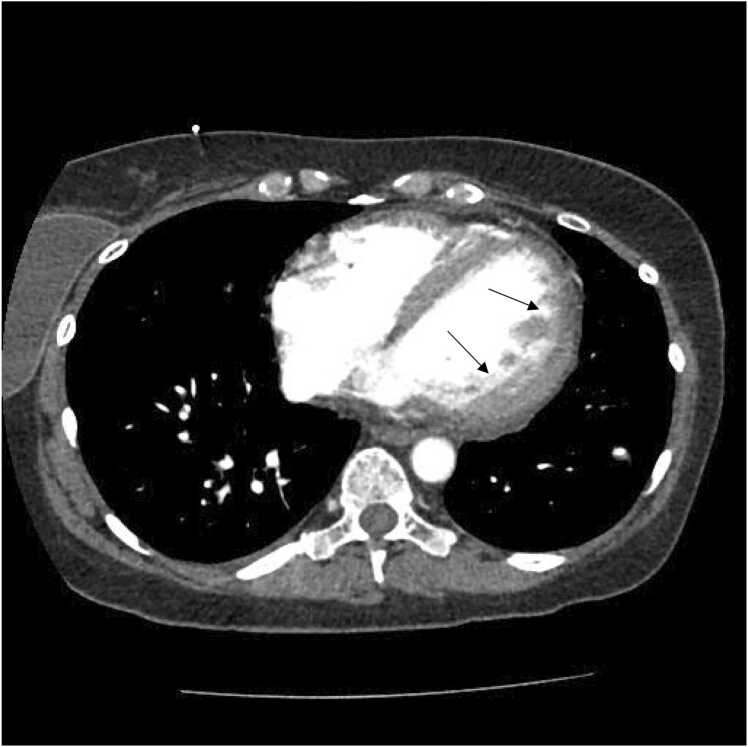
Contrast-enhanced axial CT scan showing hypertrophic ventricular trabeculations and deep interventricular recesses *(black arrows)*.
